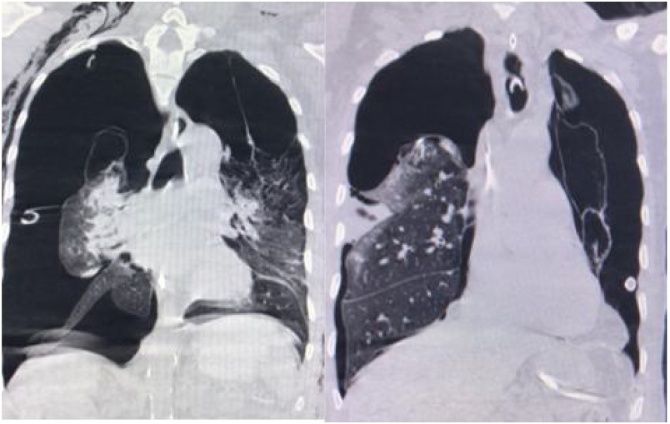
Thorax CT without contrast performed at admission on the left side, and one day after bullectomy on the right side. It demonstrates a significant improvement in pulmonary expansion after decortication of right lobe; the collapsed left side, however, was not demonstrated at time of admission to the trauma center, in a left image.
